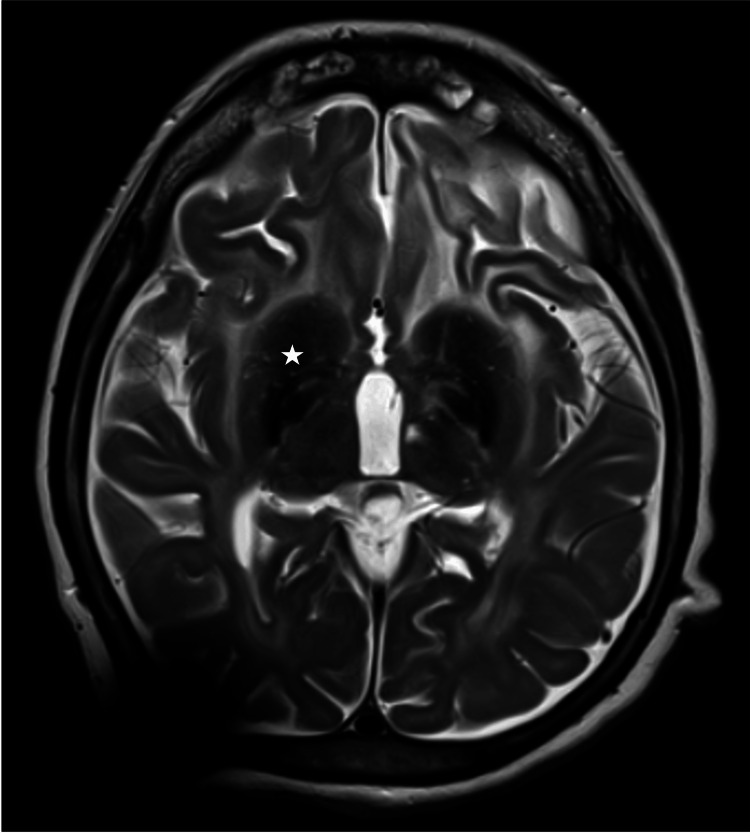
Axial T2 Weighted Imaging Axial T2-weighted image obtained at 18 years of age at the level of the basal ganglia demonstrates diffuse cerebral volume loss, with pronounced T2 hypointense signal within bilateral basal ganglia (asterix), particularly the globi pallidi. There remains relative sparing of the medial medullary laminae. Note, susceptibility artifact with resultant image distortion along the right parieto-occipital region related to ventriculoperitoneal shunt.
